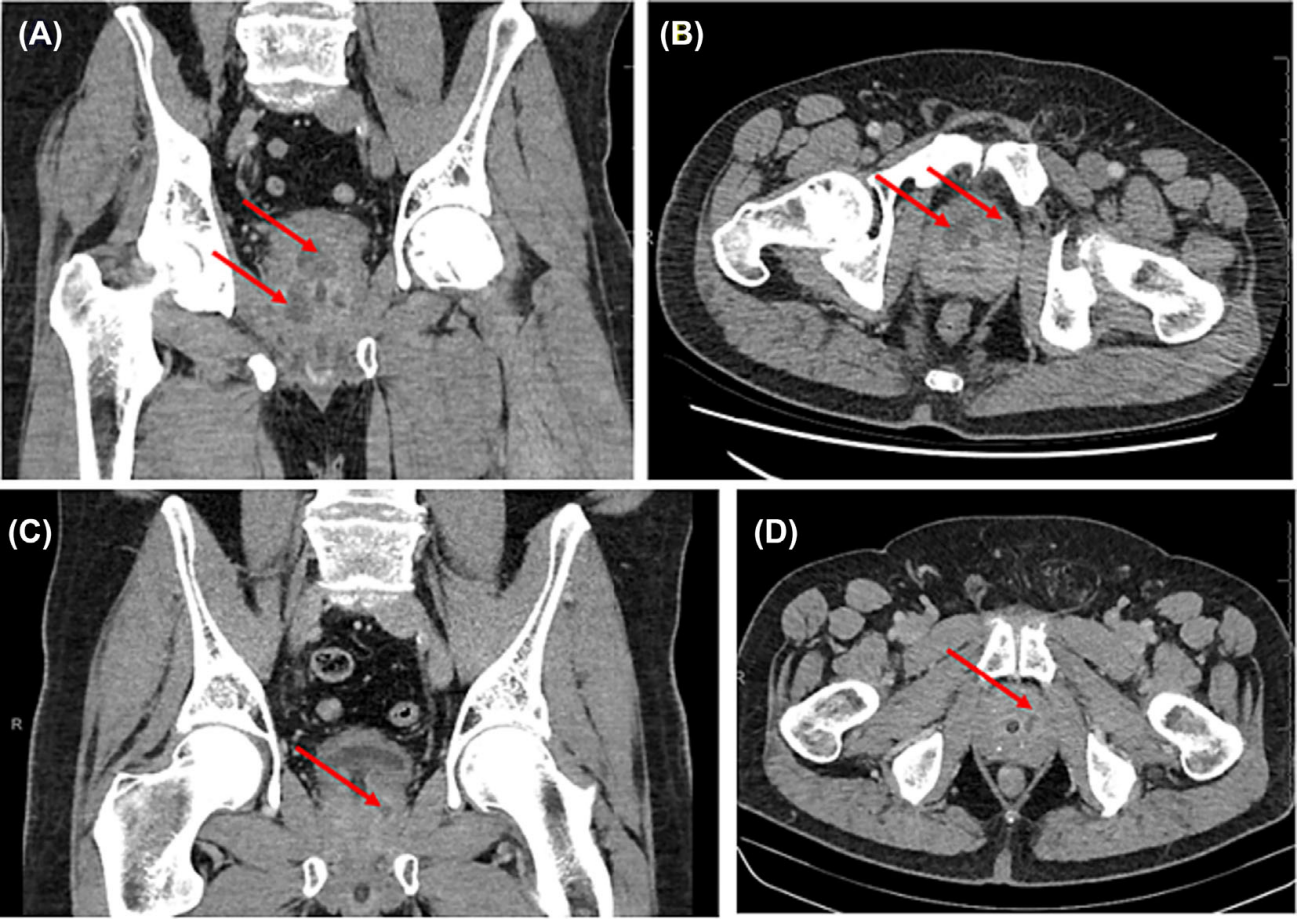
CT images showing the prostate abscess for Case 2, a 61‐year‐old male. (A) Coronal view and (B) axial view show multiple prostate abscesses. (C) Coronal view and (D) axial view were obtained 4 weeks post‐drainage and show a smaller, single 2 cm × 1 cm collection.
